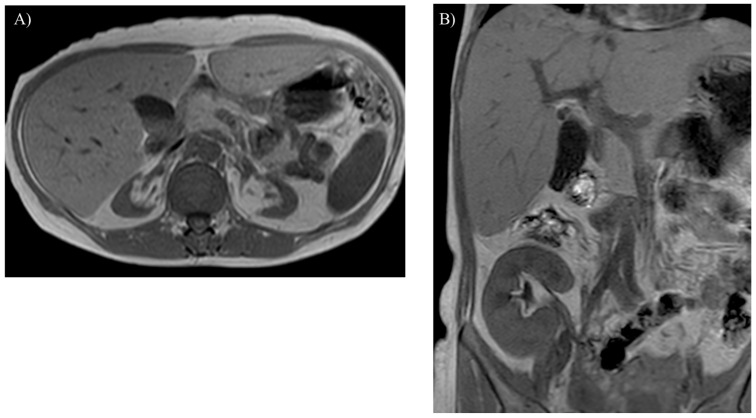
Renal atrophy is observed on T1-weighted imaging in a 40-year-old woman with glycogen storage disease type Ia. The atrophic kidneys lose corticomedullary contrast on T1-weighted imaging (A). A transplanted kidney shows normal corticomedullary contrast on T1-weighted imaging (B).
